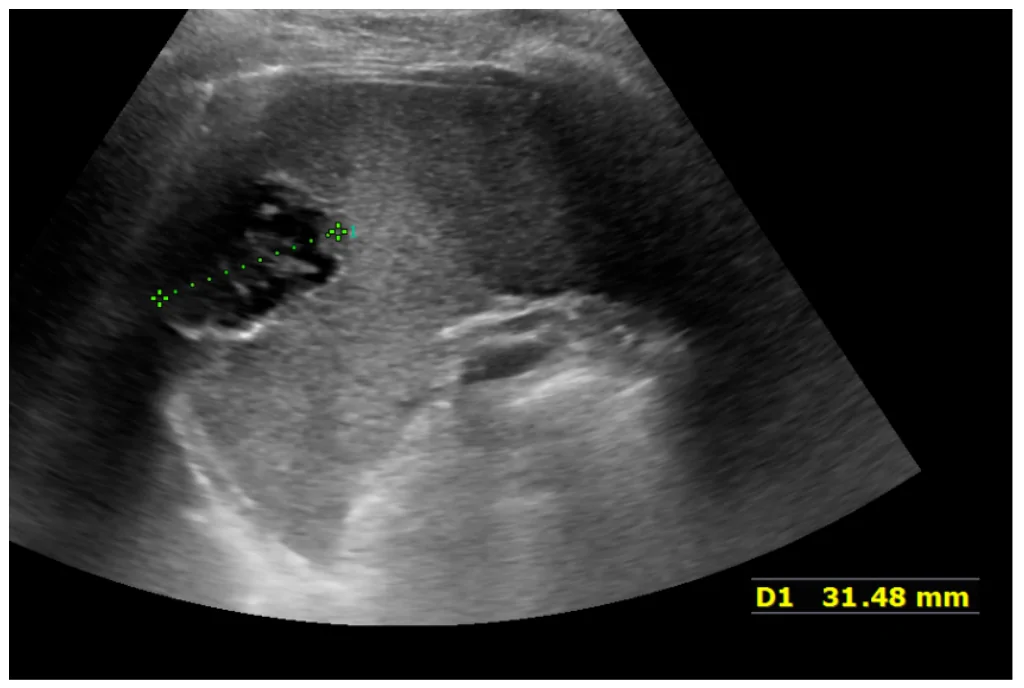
Follow-up grayscale (B-mode) splenic ultrasound (April 2024, six months after treatment). Within a normal-sized spleen with homogeneous parenchyma, a well-defined rounded lesion (calipers) is seen with a partly calcified wall and heterogeneous, predominantly solid hyperechoic content and coarse internal echogenic foci consistent with calcification, without an anechoic fluid component or detached endocyst membranes. The appearances are those of an inactive-appearing hydatid cyst (WHO-IWGE CE4–CE5).
